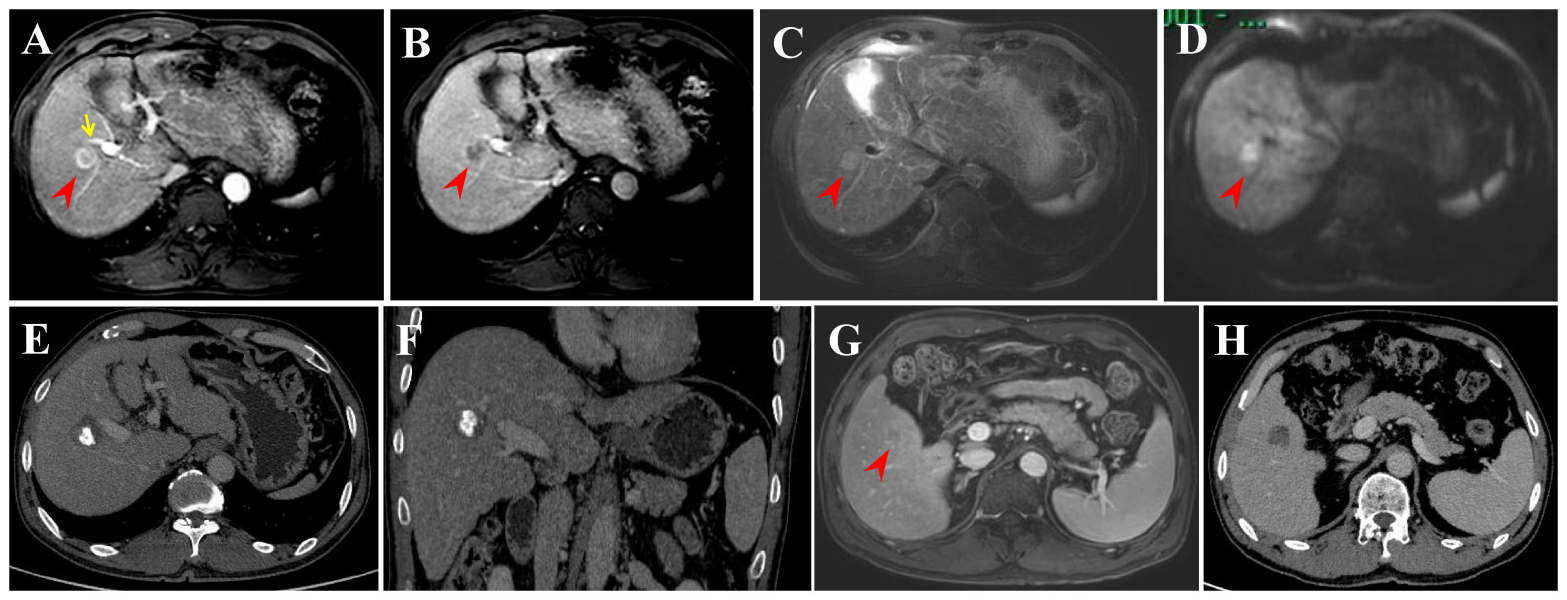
Intrahepatic distant recurrence (IDR) after transarterial chemoembolization (TACE) + radiofrequency ablation (RFA) for perivascular HCC in a 55-year-old man. (A-D) Dynamic contrast-enhanced axial magnetic resonance (DCE-MRI) scans show a small HCC (arrowheads) in periportal location (arrow) before treatment. (E, F) Contrast-enhanced computed tomography (CECT) scans obtained during portal venous phase 1 month after TACE + RFA showing the complete ablation zone adjacent to the portal vein. (G) DCE-MRI scans obtained during hepatic arterial phase (not shown) and portal venous phase 31 months after TACE + RFA showing the IDR (segment V). (H) CECT scan obtained during portal venous phase 1 month after RFA showing the complete ablation zone.
